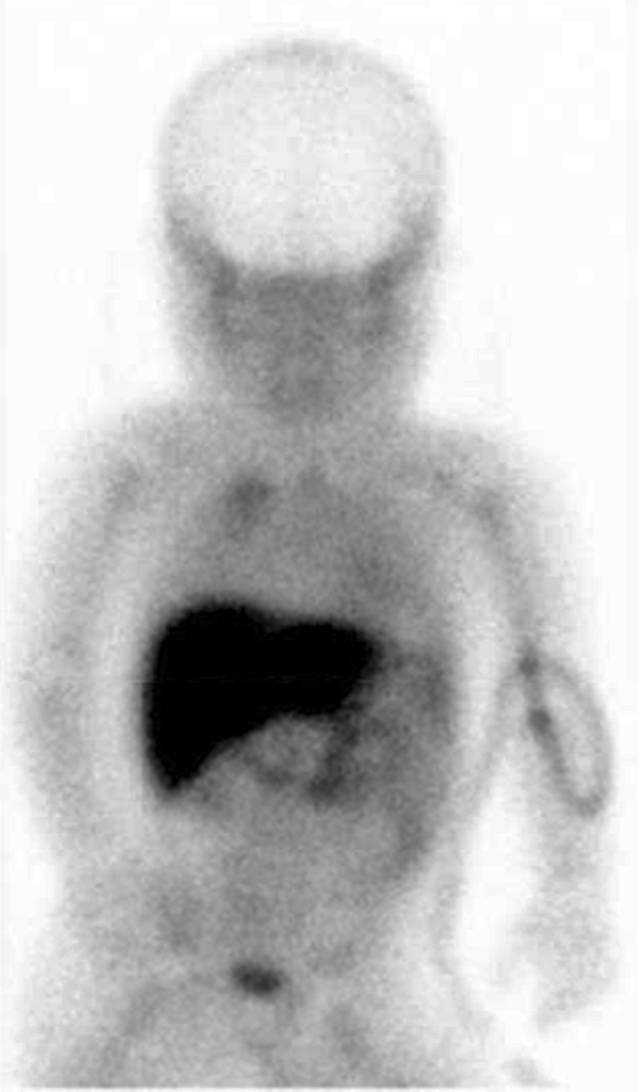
In der Praxis der Hirntoddiagnostik, die der Autor seit über 20 Jahren aus persönlicher Erfahrung überblickt, liegt heute bei allen betroffenen Patienten mindestens eine Schnittbildgebung (CT, MRT) des Gehirns vor, um den richtliniengemäßen Nachweis der schweren ausgedehnten Hirnschädigung zu führen (Ausnahmen sind möglich bei offensichtlicher Ursache, wie z. B. schwerster Schädelquetschung). Behebbare Ursachen des Hirnfunktionsausfalls werden ausgeschlossen (hirntodähnliche Erkrankungen, Medikamentenwirkungen, Stoffwechselentgleisungen usw.; [1, 35, 36]). Wenn Zweifel in Bezug auf eine medikamentöse Beeinflussung verbleiben, kommt ein Verfahren zur Feststellung des zerebralen Zirkulationsstillstandes zum Einsatz [35]. Dies gilt auch dann, wenn ein Hirnstammreflex oder der Test auf Atemstillstand nicht zuverlässig durchführbar ist [36]. Wenn an der Hirntoddiagnose auch nur geringste Zweifel bestehen, wird der Hirntod nicht festgestellt, sondern die Hirntoddiagnostik nach einem geeigneten Zeitintervall vollständig wiederholt oder ansonsten abgebrochen [3].
